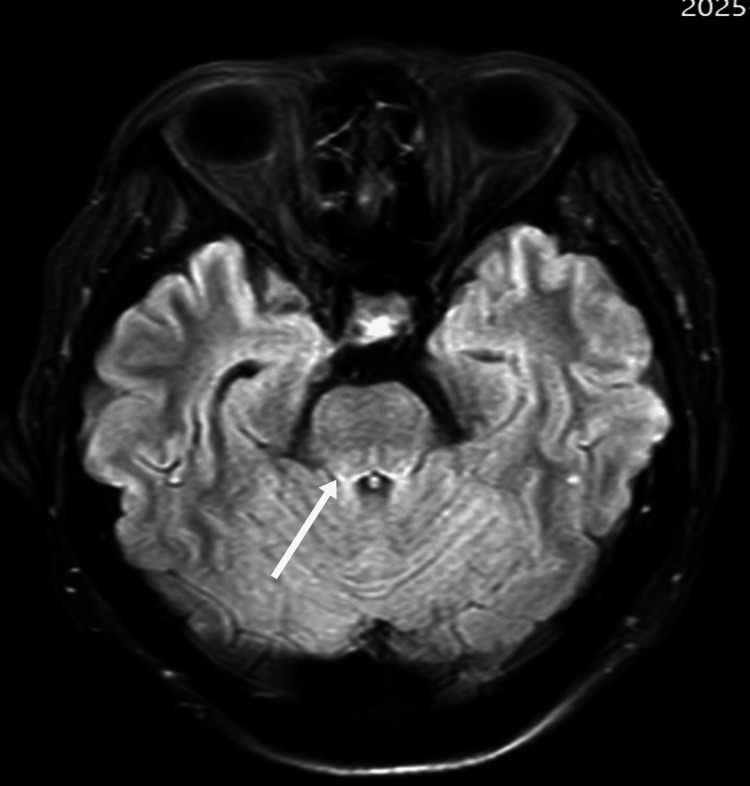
Axial FLAIR MRI of the cerebral peduncle after treatment Follow-up axial T2-FLAIR image demonstrates marked resolution of the previously noted hyperintense lesion in the cerebral peduncle. As indicated by the white arrow, there is a reduction in the abnormal signal of the cerebral peduncle FLAIR: fluid-attenuated inversion recovery; MRI: magnetic resonance imaging
